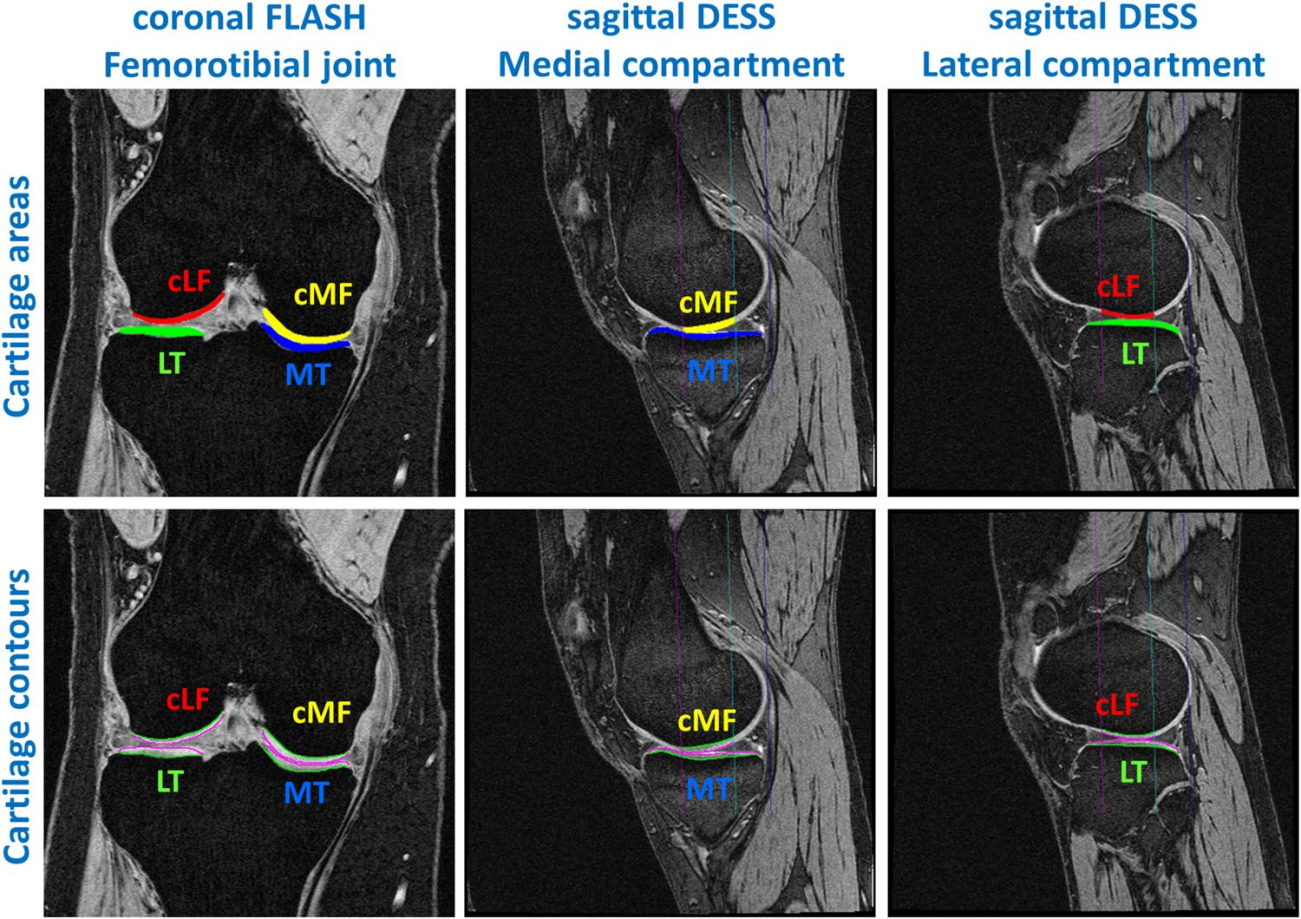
Manual segmentation of the femorotibial cartilages [*MT/LT* medial/lateral tibia, *cMF/cLF* central (weight-bearing) part of the medial and lateral femoral condyles] from coronal FLASH and sagittal DESS MRI. The figure shows the cartilage areas (top row) and the cartilage contours (bottom; green: total area of subchondral bone; magenta: cartilage surface area). The sagittal MRIs also show the 60% femoral region of interest (magenta line: anterior margin; blue line: posterior end of the condyles; turquoise line: 60% margin)
